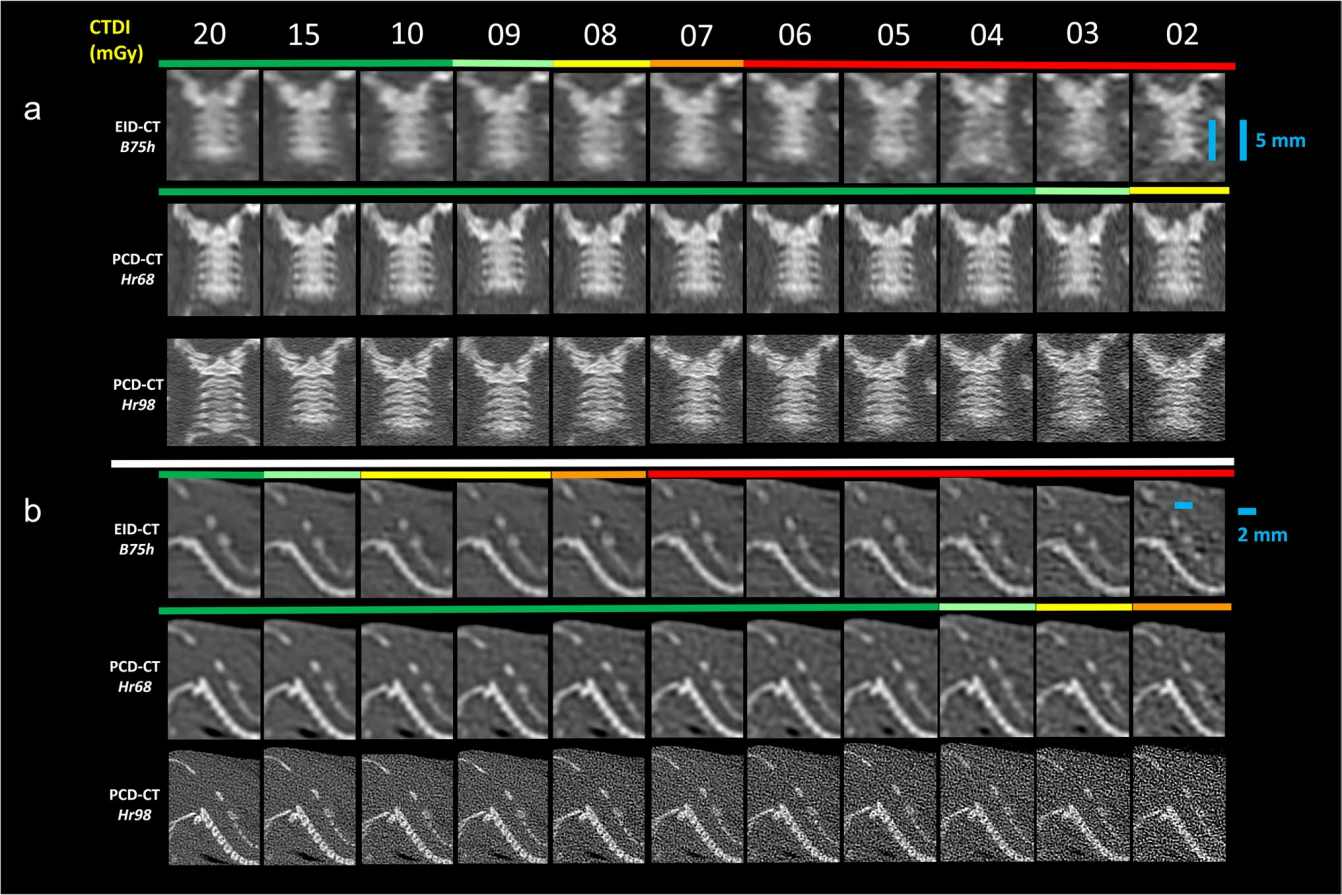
Semiquantitative evaluation of bone detail visualization on 0.6-mm coronal and 0.2-mm sagittal reformations of the cervical spine. (a) 0.6-mm coronal reformations of the cervical spine for the visualization of the transverse processes. (b) Sagittal 0.2-mm reconstructions of the cervical spine for visualization of the intervertebral discs. Colored lines indicate semiquantitative evaluation of two readers in consensus for delineation of transverse processes (a) and intervertebral discs (b) at various CTDI_Vol_ values; red, no delineation; yellow, unsharp delineation; green, sharp delineation. CTDI_Vol_, volumetric CT dose index; EID-CT, energy-integrating detector CT; PCD-CT, photon-counting detector CT; B75h, Hr68, and Hr98 are abbreviations for specific reconstruction algorithms
